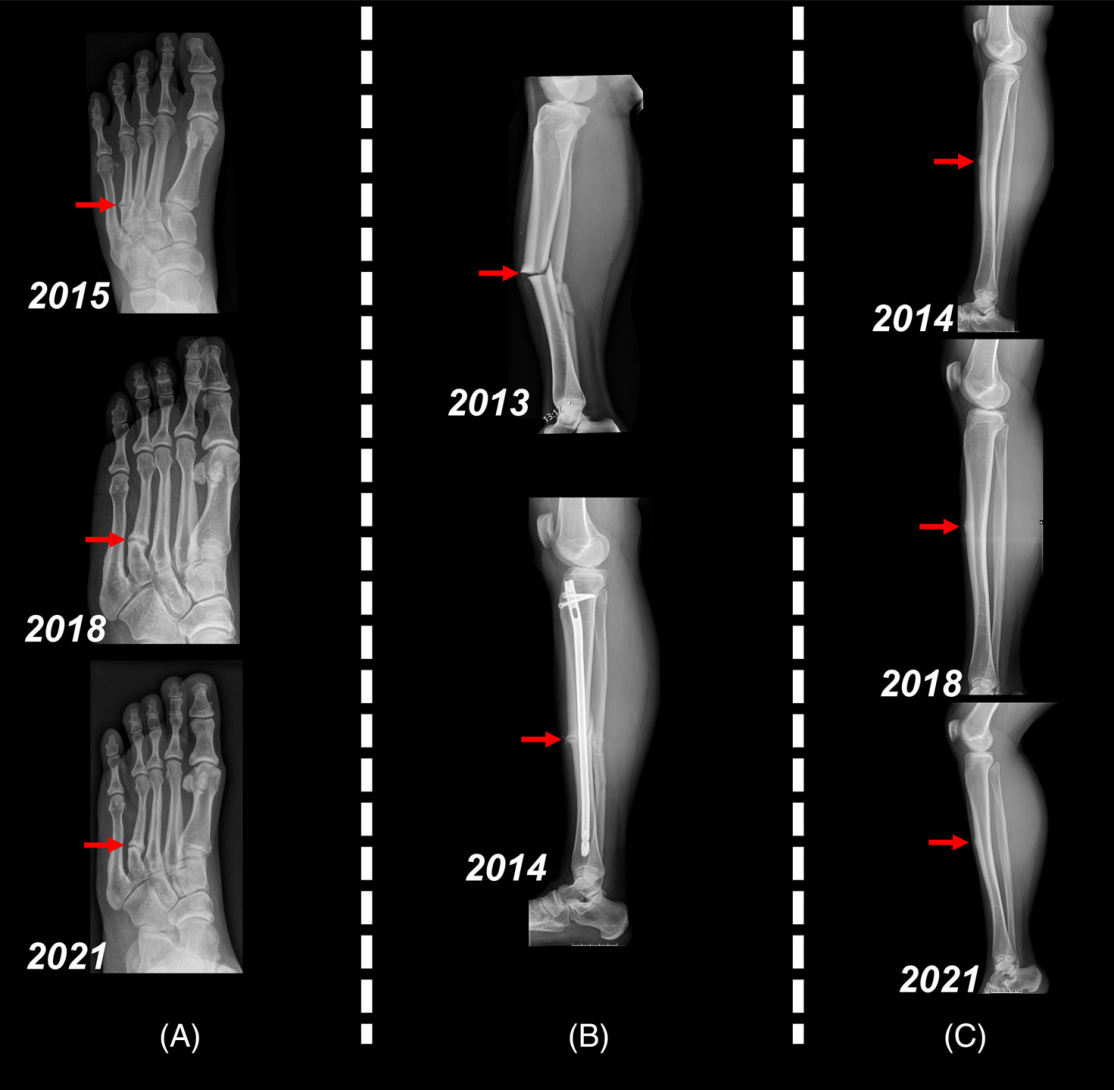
Radiographs of the left proximal fourth metatarsal (*A*), right tibial diaphysis and fibula (*B*), and left tibial diaphysis (*C*) showing fatigue fractures and delayed healing (indicated in red).
